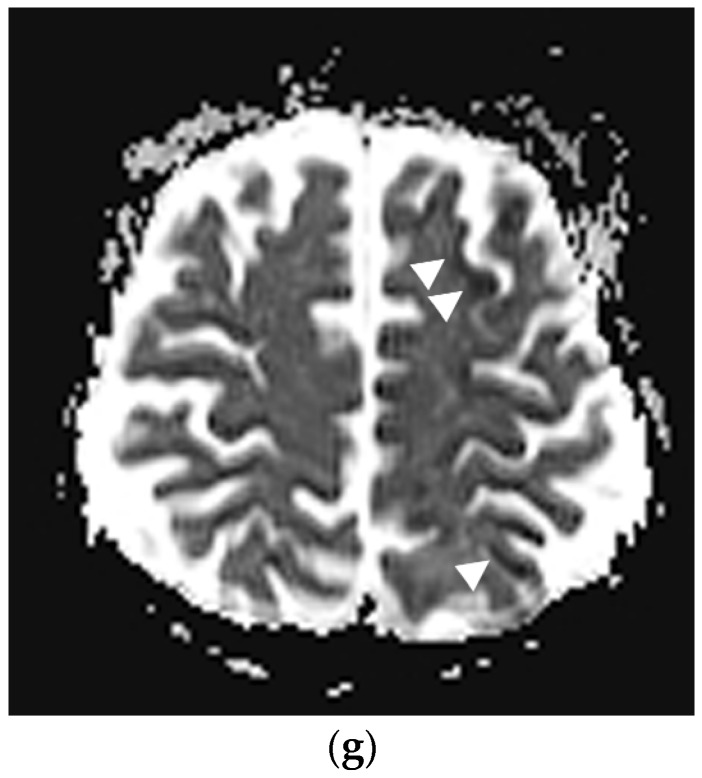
Following the placement of right internal jugular tunneled Hickman line, a 76-year-old man developed an episode of desaturation, tachycardia, and non-responsiveness. Subsequently, an immediate CT of brain, neck and chest was performed and gas was found in the left cavernous sinus (a,b) and within a left parietal sulcus (c); it was felt to represent air in a vessel, possibly a pial vein. There was also a large amount of air surrounding the right internal jugular vein (d); with a partially visualized central venous catheter (*). A brain MRI was performed showing FLAIR hyperintensity in multiple regions (e) with evidence of restricted diffusion (f); and corresponding low signal (arrowheads) on the apparent diffusion coefficient (ADC) images (g). The patient received a single treatment of hyperbaric oxygen but died 8 days later.
